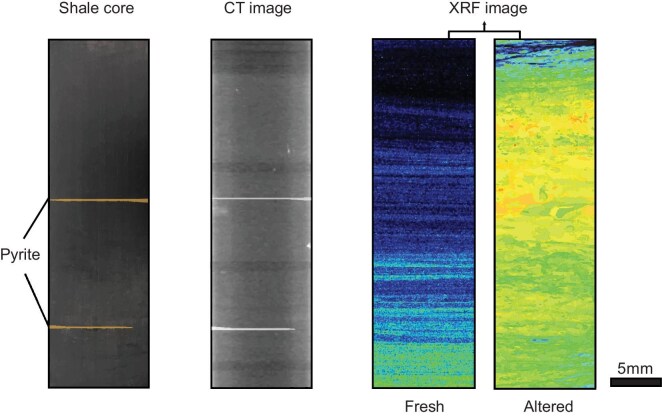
An imaging-guided approach for shale Re–Os geochronology. CT and XRF scanning can be used to aid in selecting the best-preserved shale intervals for Re–Os geochronology akin to cathodoluminescence imaging used in zircon U–Pb geochronology. The shale core and CT image are from the Lantian biota [36]. The Sr distribution maps obtained by XRF are cores from the Qingjiang biota.
